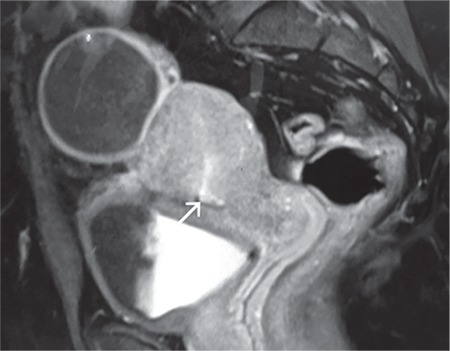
Post-surgical magnetic resonance image after 2 months, showing the LSCS scar (arrow) with no tract demonstrable. Additionally, she developed a simple ovarian cyst, which resolved on its own in 8 weeks LSCS: Lower segment cesarian section
